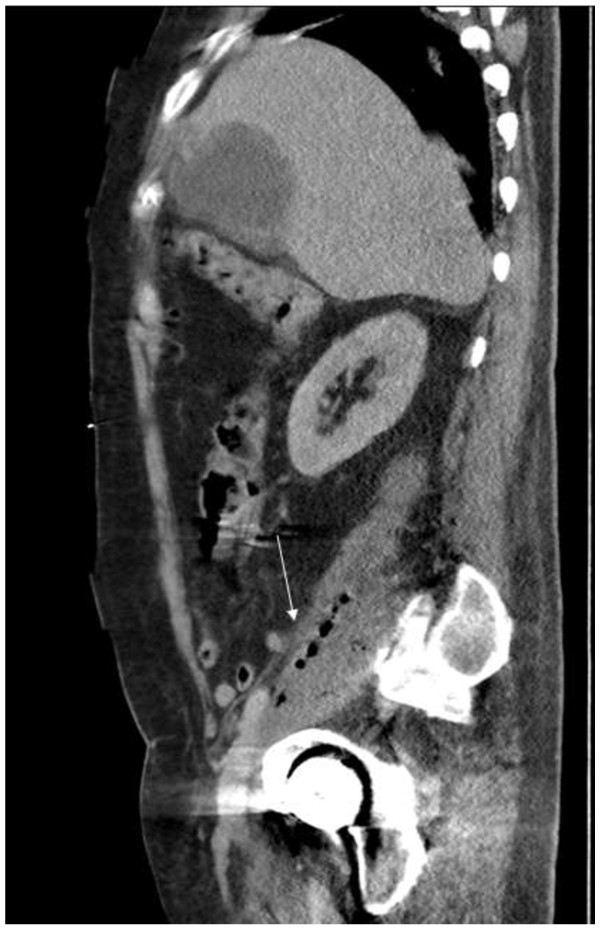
CT demonstrated Sagittal View of Abdomen and Pelvis demonstrating gas locules in Right Iliopsoas Region.
